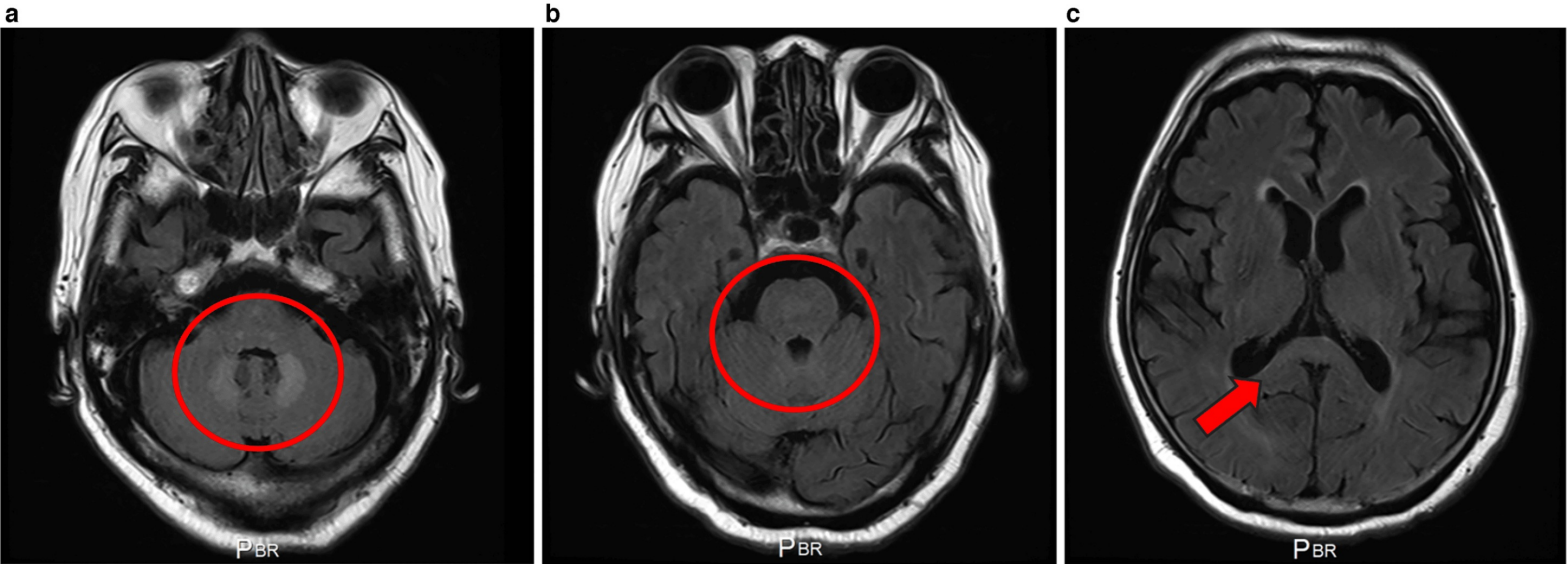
Initial brain MRI on day 63 of metronidazole therapy Axial FLAIR images show symmetric hyperintensities in the cerebellar dentate nuclei (a; red circles) and dorsal midbrain tegmentum (b; red circles), with a faint lesion in the splenium of the corpus callosum (c; single red arrow). The bilateral, posterior fossa-predominant distribution is characteristic of metronidazole-induced encephalopathy. FLAIR: fluid-attenuated inversion recovery; MRI: magnetic resonance imaging
